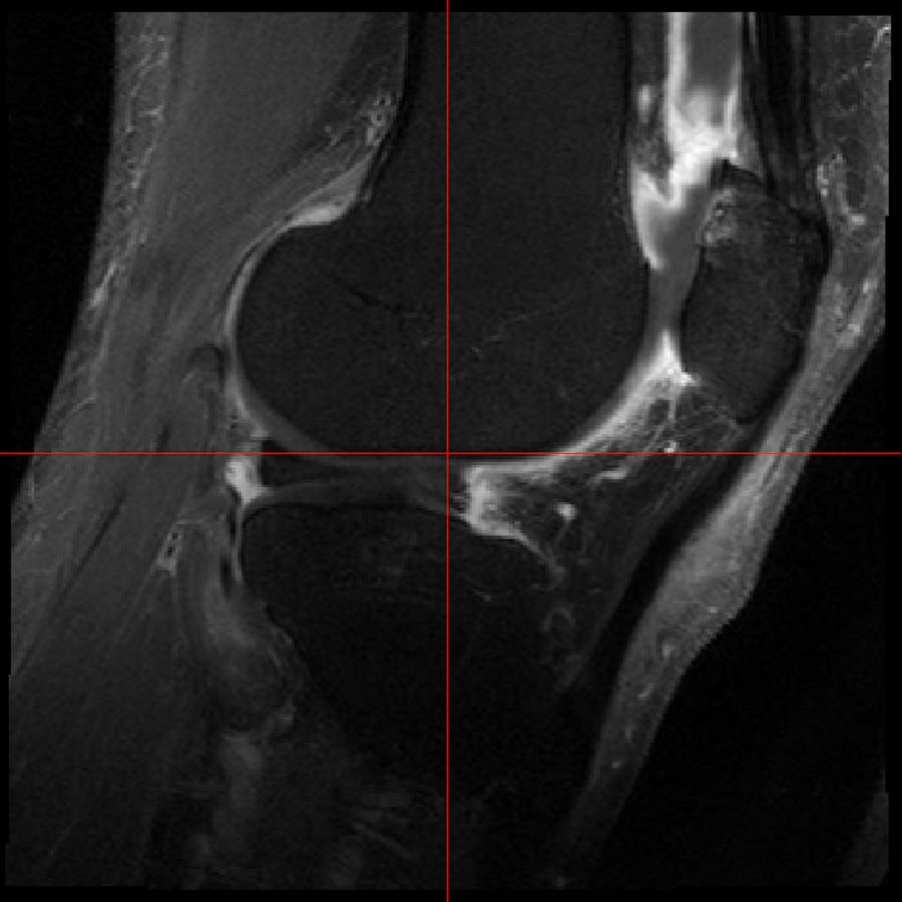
Gadolinium-enhanced MRI of study patient, showing synovial tissue and fluid differentiation.
